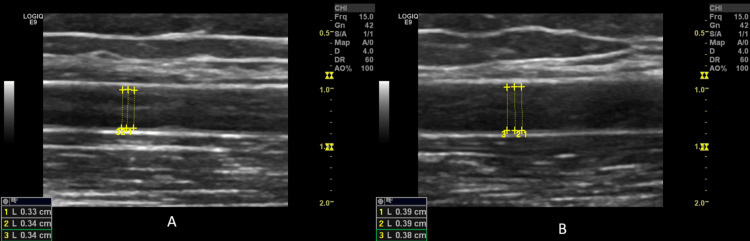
Longitudinal ultrasound images of the brachial artery showing placement of calipers for diameter measurement before (A), and 45-60 seconds after the deflation of the cuff (B). Endothelium-dependent flow-mediated dilatation; the endothelium-dependent vasodilation response was 5%.
